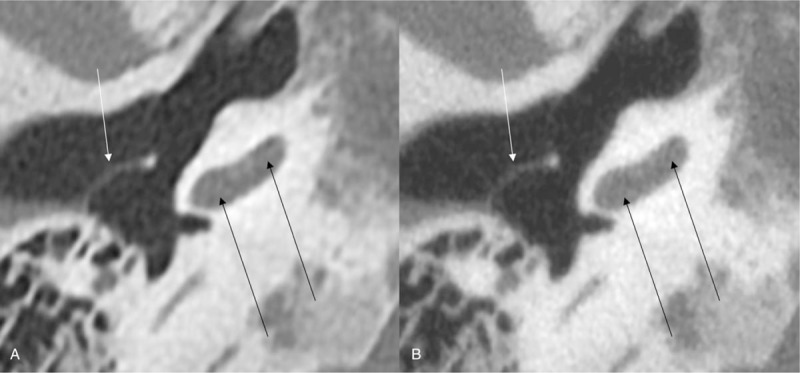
Temporal bone CT in a second patient reconstructed with hybrid iterative reconstruction (HIR) (A) and with model-based iterative reconstruction (MBIR) (B). The spiral osseous lamina is well-delineated in HIR (A; black arrows; score 3), but nearly invisible in MBIR (B; black arrows; score 1). The tympanic membrane is well-delineated both in HIR (A; white arrow; score 3) and MBIR (B; white arrow; score 1). Two radiologists graded both structures in an identical manner in this case.
